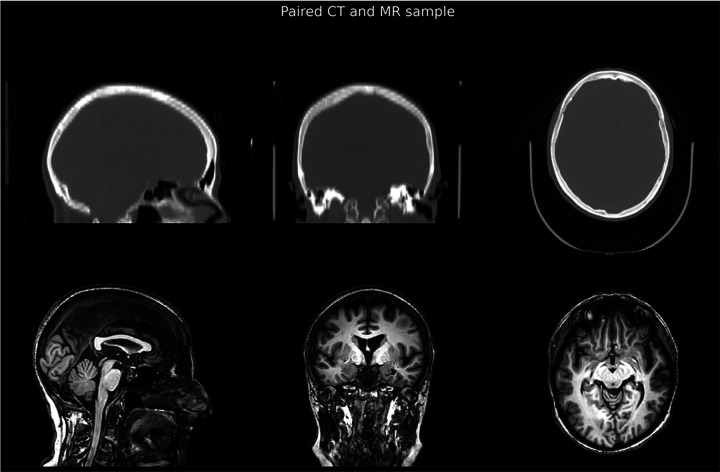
Example of Paired CT and MR images
